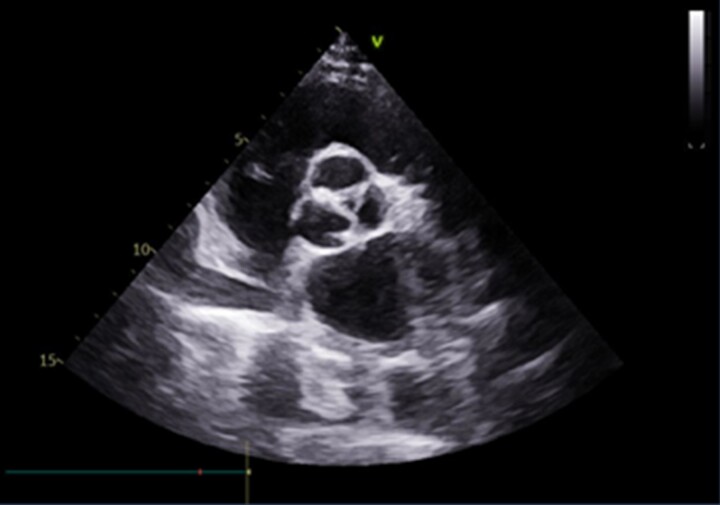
Tricuspid severely stenosed calcified aortic valve on echocardiographic examination.
